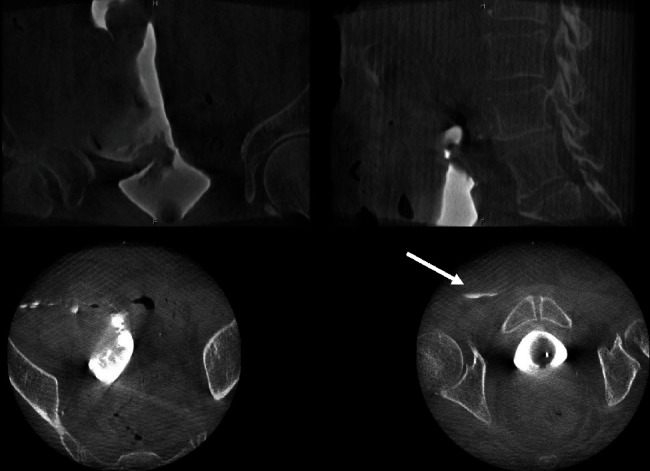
Intraoperative CT cystogram obtained using an O-arm™ surgical imaging system with three-dimensional volumetric reconstruction. Image acquisition in the coronal (top left), sagittal (top right), axial abdomen (bottom left), and axial pelvis (bottom right) planes was obtained. Contrast is visible in the intraperitoneal cavity and around the bowel, confirming the diagnosis of an intraperitoneal bladder perforation. Note the extravasation of contrast into a right inguinal hernia in the axial pelvis plane, also confirming an intraperitoneal bladder perforation.
